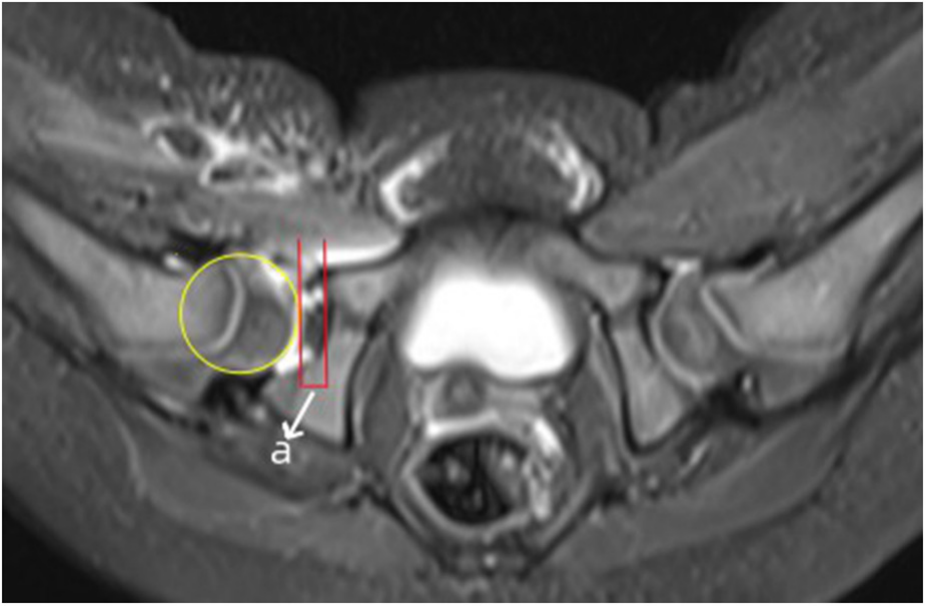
Measurement of aAFD (in mm) on an axial MR image. aAFD displaying the maximum diameter of the femoral head of a hip after CR and spica casting. The letter ‘a’ represents aAFD, which is the distance between the acetabular edge and the femoral head edge at the level of the femoral head center.
